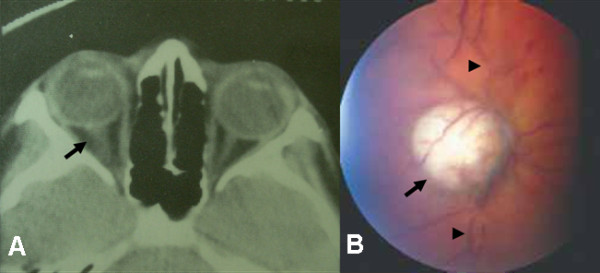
Clinical presentation and computed tomography images of a 12-year-old Caucasian boy. (A) His right optic nerve appears elevated and enhanced on computed axial tomography (arrow). His left optic nerve appears normal. (B) A well-defined whitish mass at the optic disc (arrow) with tortuous and dilated retinal vessels (arrowhead) by fundoscopy.
